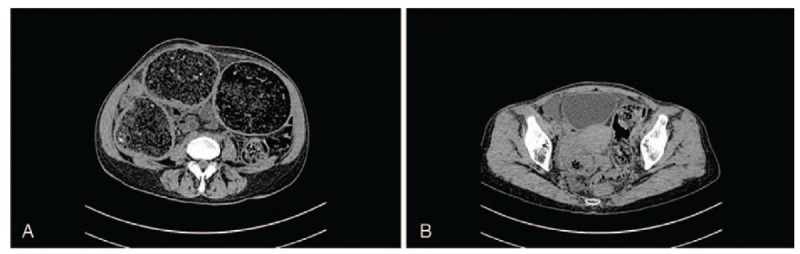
Computed tomography: dilatation of the colon above the sigmoid colon.
